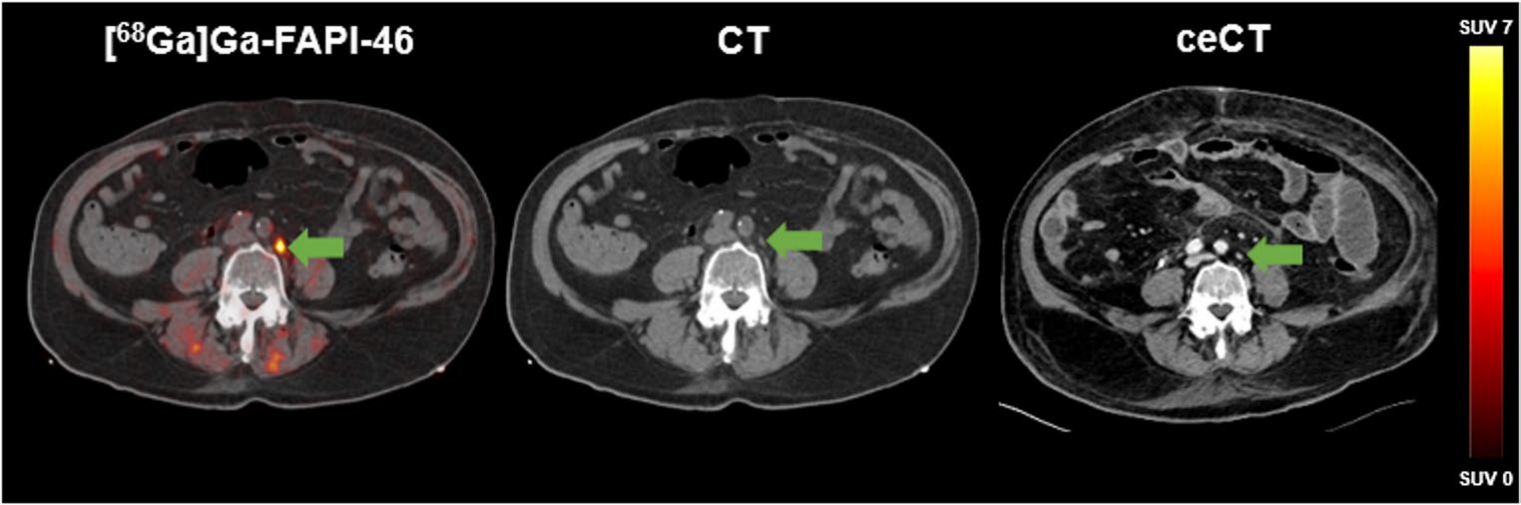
[^68^Ga]Ga-FAPI-46 PET/CT of a 84-year-old male patient with metastatic UC (LN). The arrow highlights a left sided paraaortic FAP-positive lymph node (SUV_max_ 16.6), The ceCT imaging showed no suspicious lymph node according to common CT criteria (short axis diameter: 0.8 cm)
